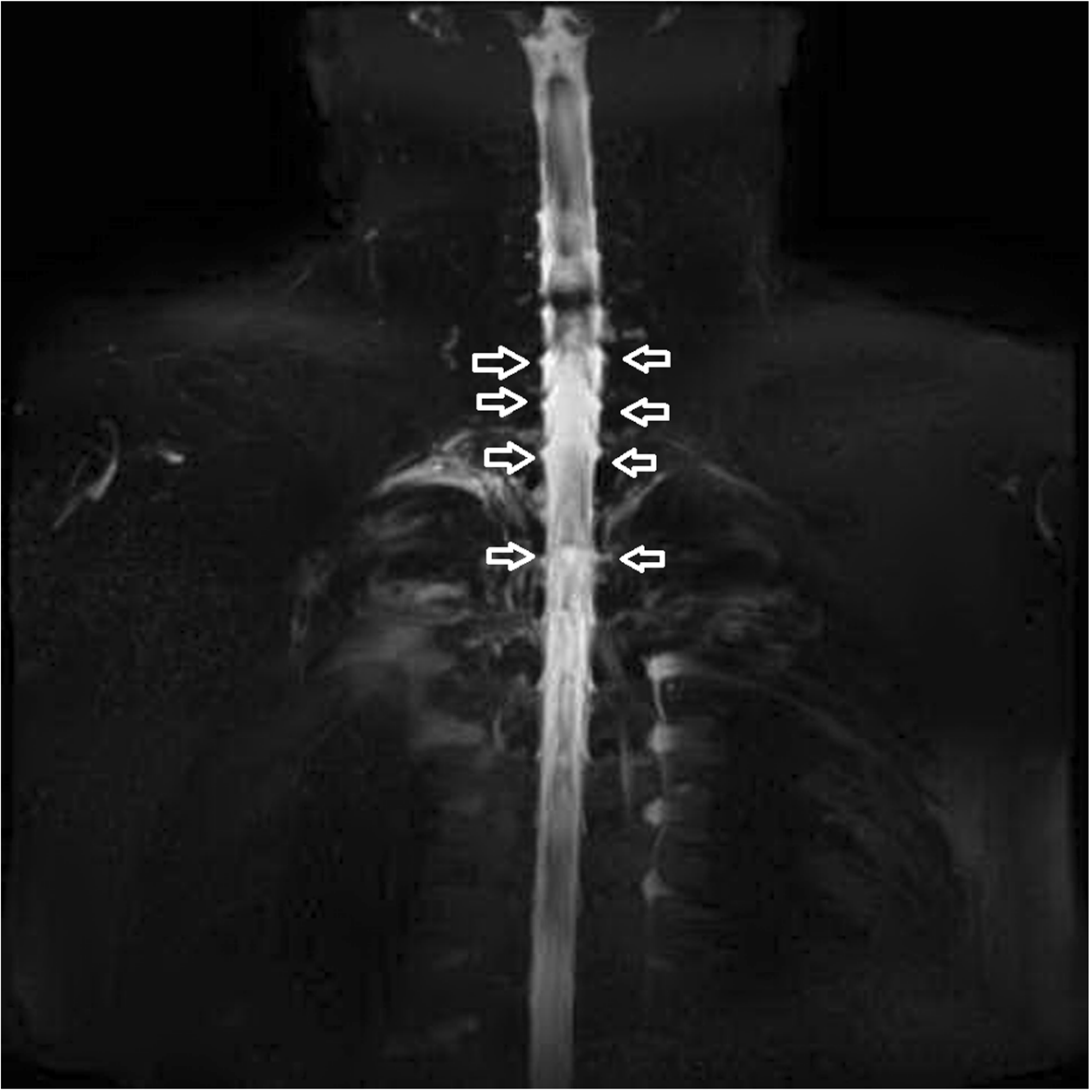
Many CSF leak lesions of lower C-and upper T spine were found using thin-slice axial multi-planar reconstruction MRM in T2-weighted three-dimensional sampling perfection with application-optimized contrasts using different flip-angle evolutions pulse sequence. Arrow: CSF leak lesions
